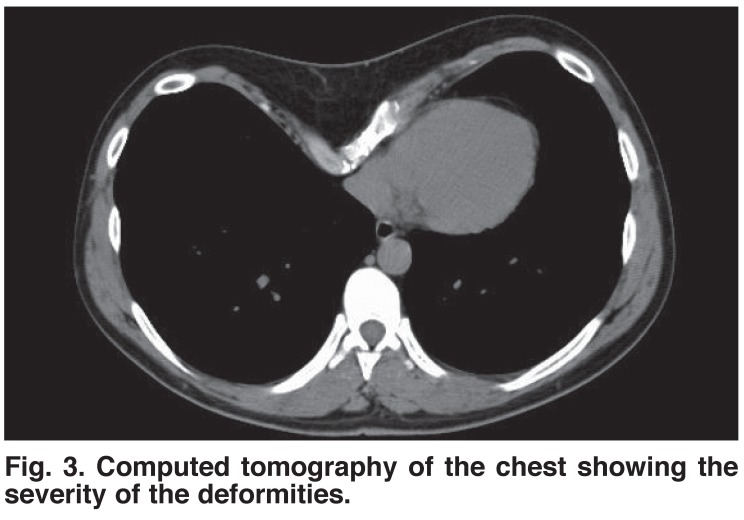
Computed tomography of the chest showing the severity of the deformities.
